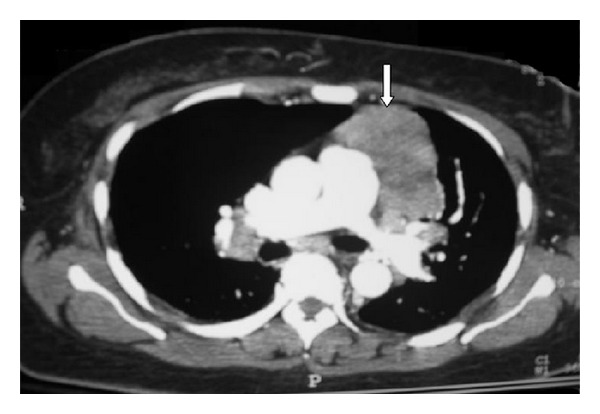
CT chest image in a patient with MG revealing large necrotic mass in the left anterior mediastinum (white arrows) and bilateral hilar lymphadenopathy (with permission from Kurukumbi et al. [5]).
